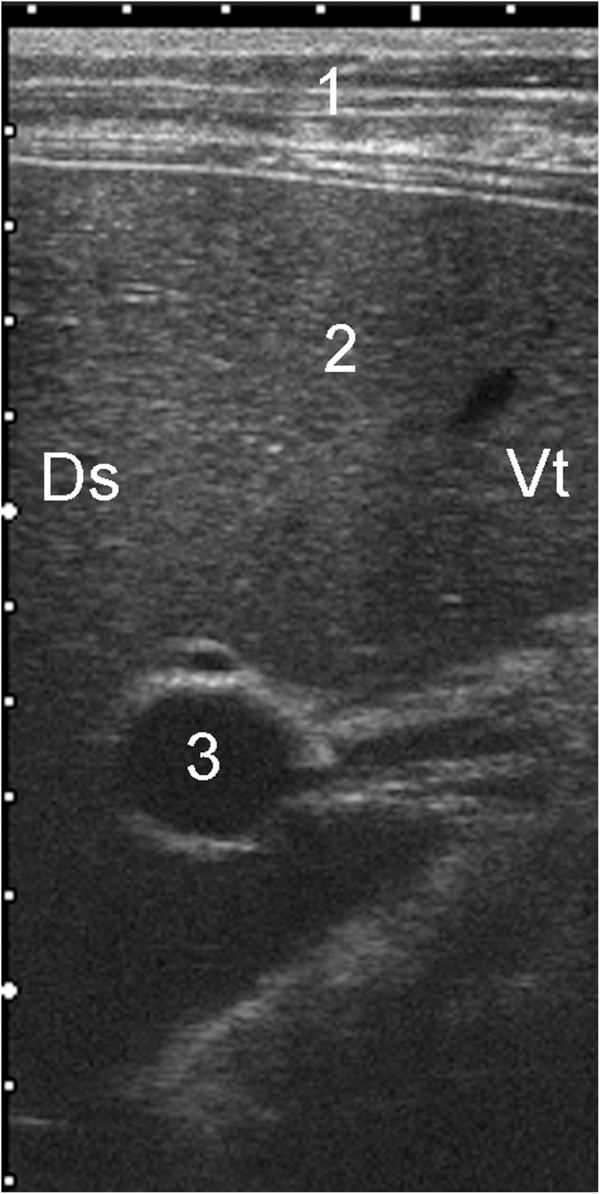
Ultrasonogram of the portal vein. Ultrasonogram of the portal vein viewed from the right in an 83-day old Holstein-Friesian calf. 1 Abdominal wall, 2 Liver parenchyma, 3 Portal vein, Ds Dorsal, Vt Ventral. (depth of the image = 12 cm).
